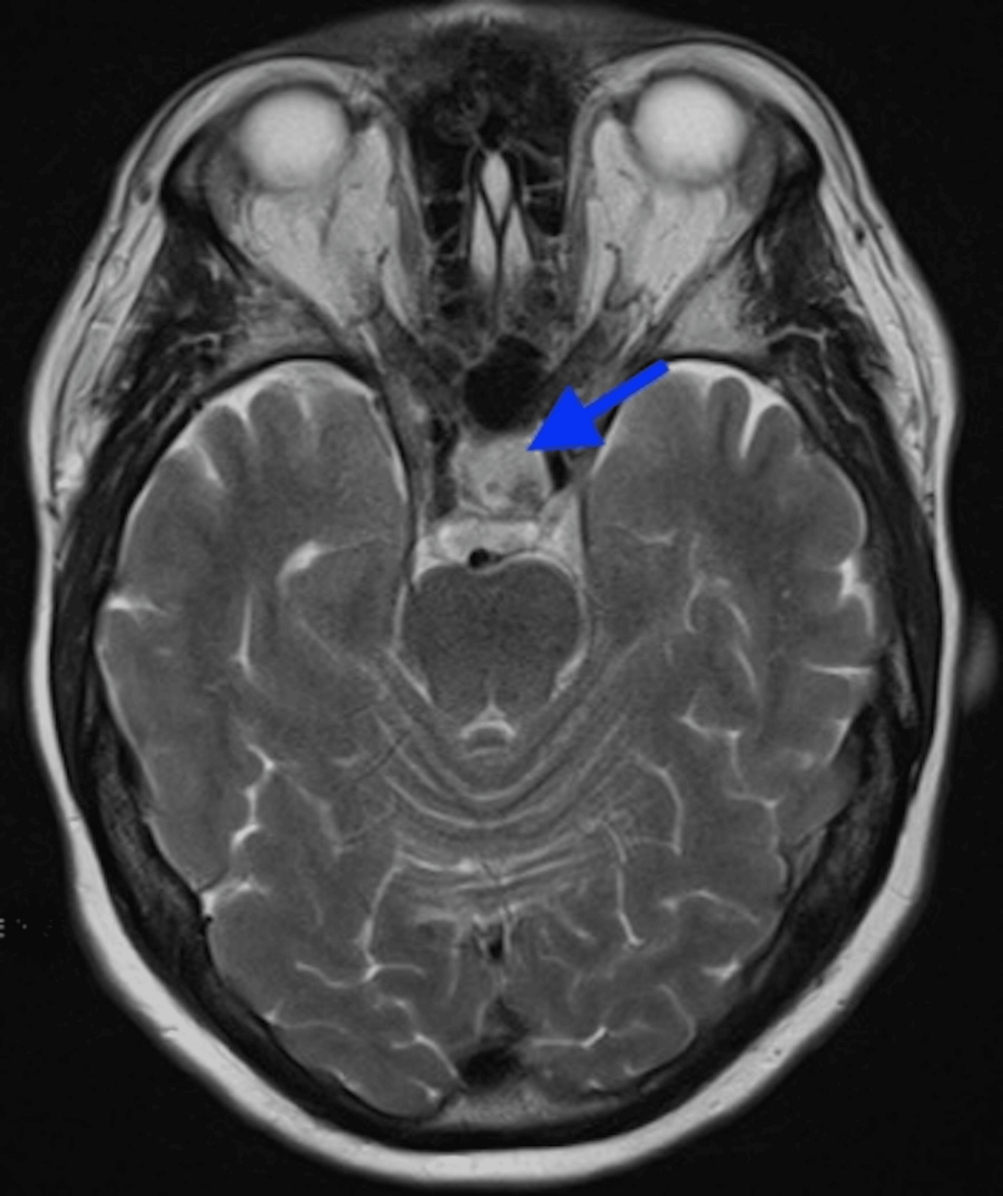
Brain MRI with pituitary protocol showed a new macroadenoma in the left pituitary moiety (marked by an arrow), measuring up to 1 cm
